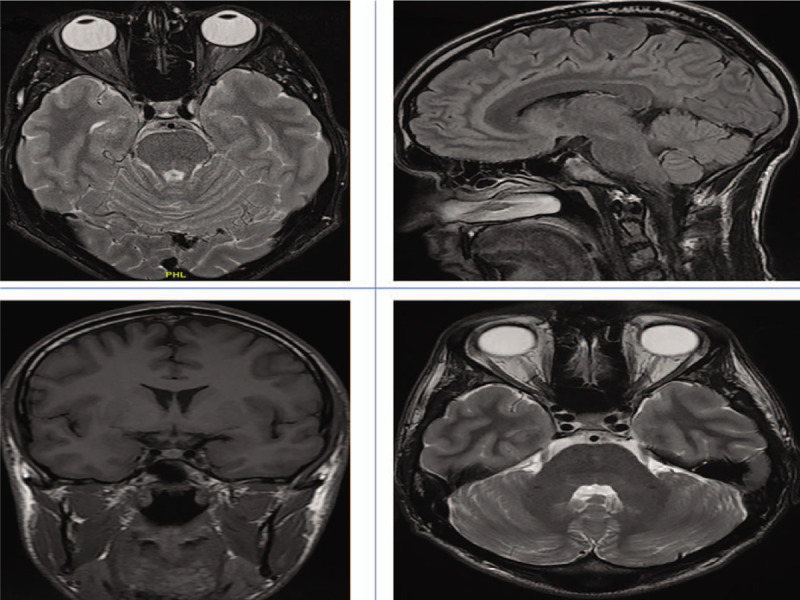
Cumulative Image of magnetic resonance imaging scan: Top row: A) Transverse section B) Sagittal section. Bottom row: C) Coronal section D) Transverse section. All sections revealed no structural abnormalities.
